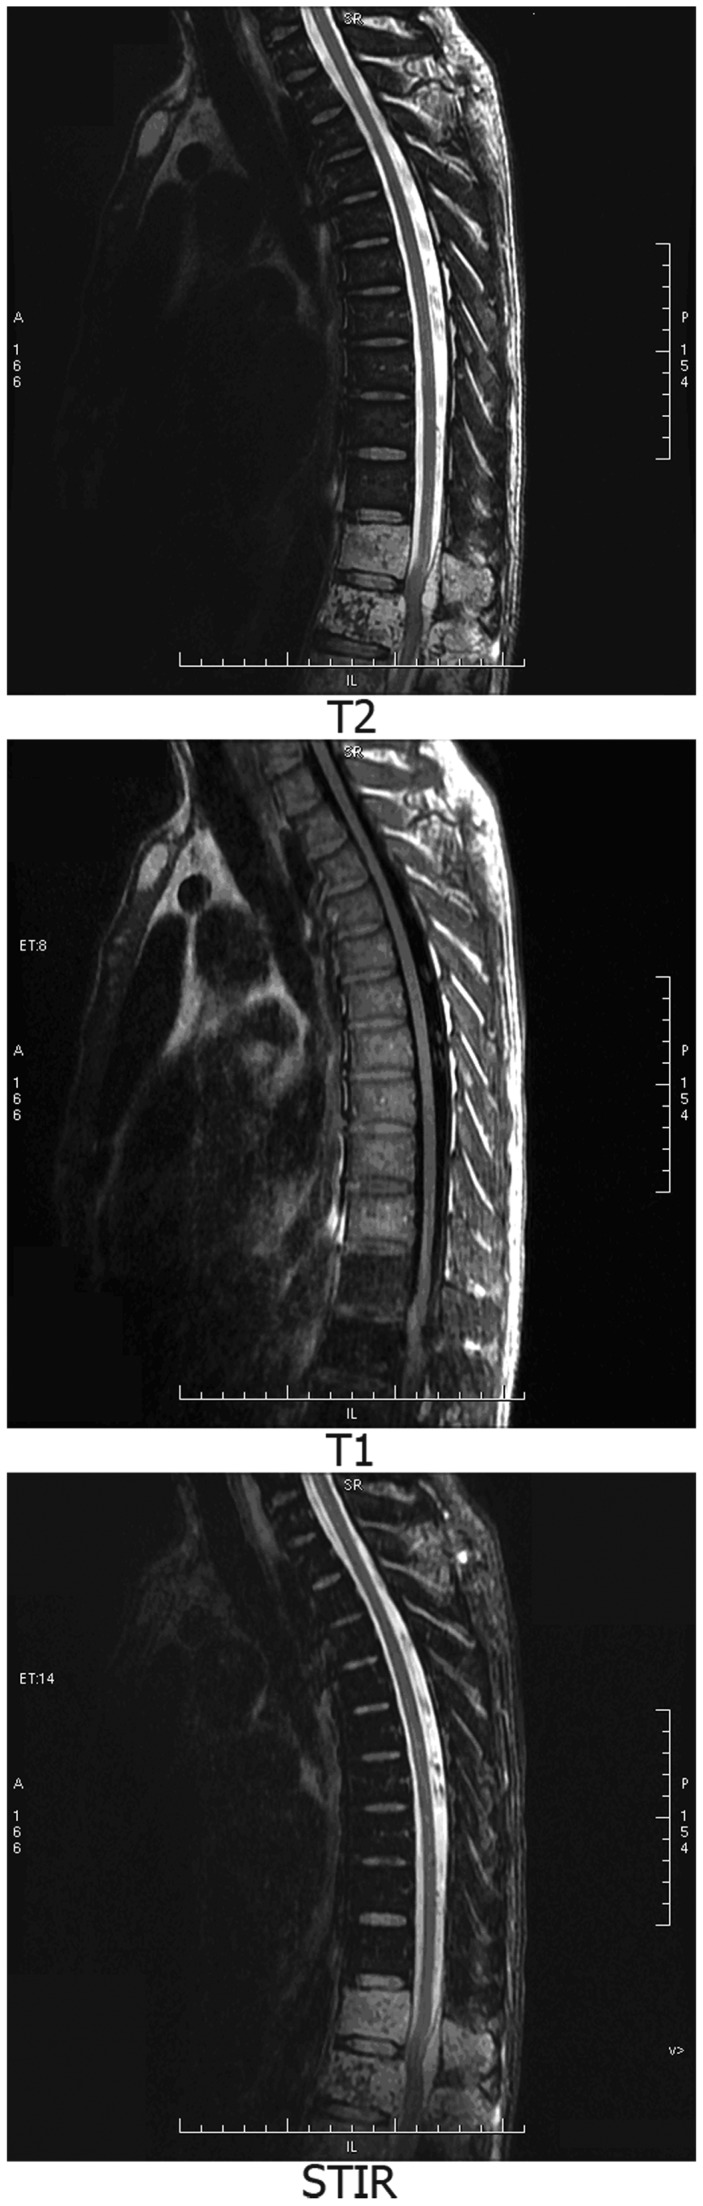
Thoracic vertebrae magnetic resonance imaging (MRI) scan showing abnormal signals in the 3rd and 4th thoracic spinous processes and the posterior soft tissues. STIR, short T1 inversion recovery.
